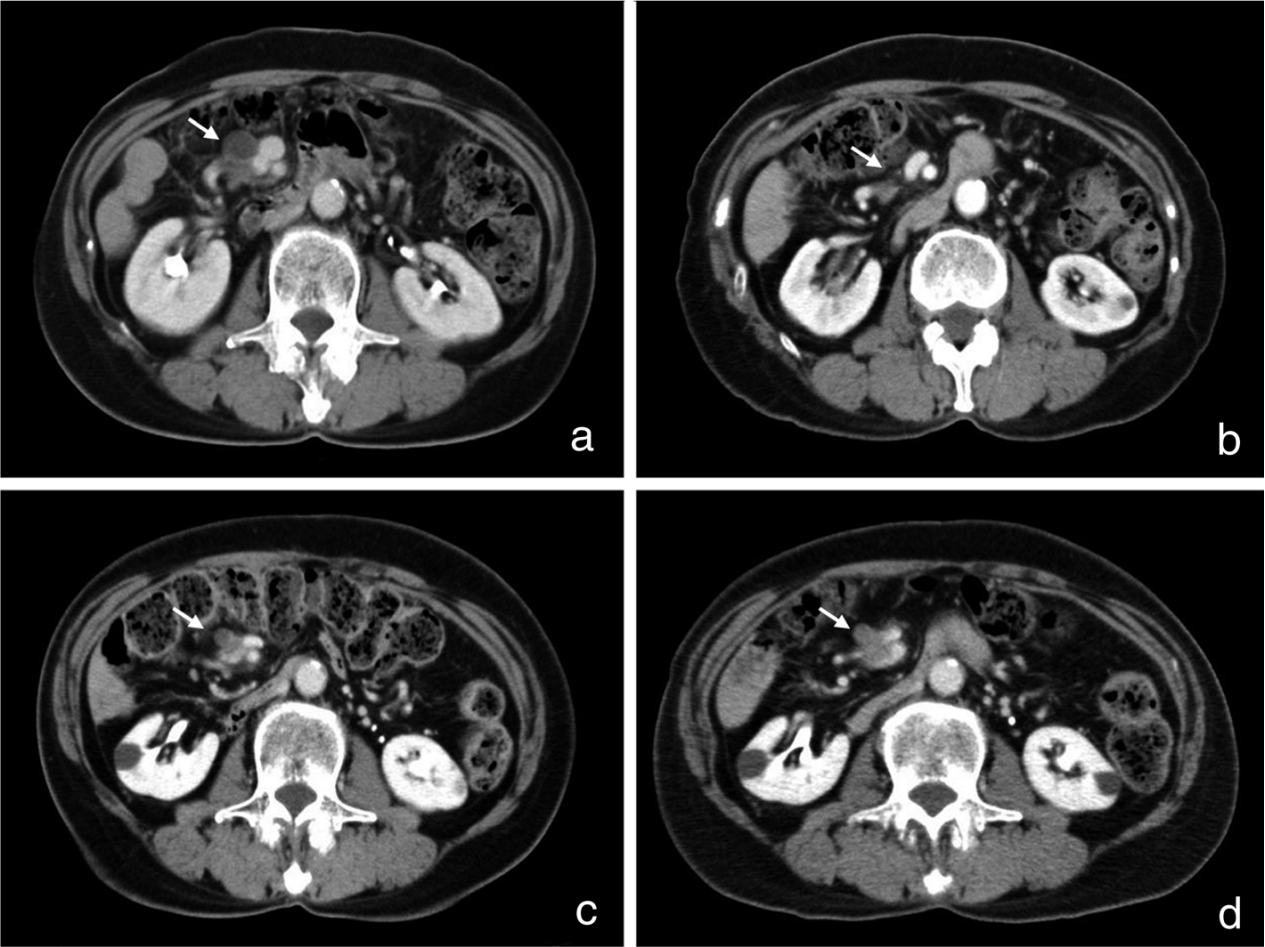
Low-density mass around SMA with chemotherapy. (a) CT examination revealed a low-density area around the SMA in 2004. (b) The tumor disappeared gradually over 3 years (to 2007). (c) Routine CT examination showed a low-density area around the SMA on August 2010. (d) The tumor was within the stable disease range after 4 months of gemcitabine treatment. Arrows show the low-density area around the SMA, which appeared after the first operation.
